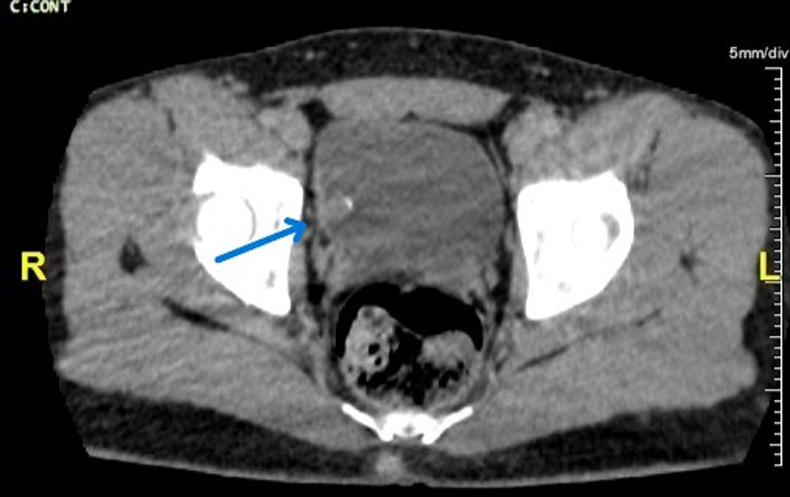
computed tomography scan confirming a hypervascular soft tissue lesion without locoregional extension or impact on the upper urinary tract
